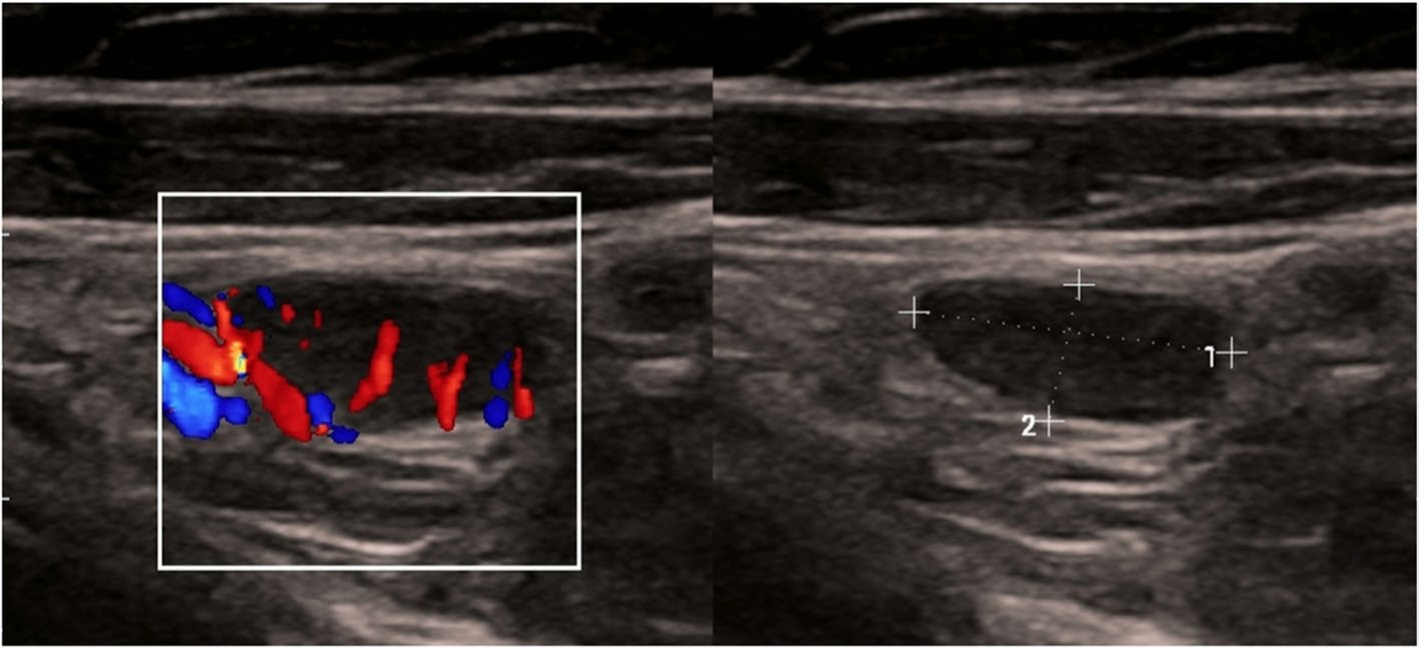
Grayscale ultrasound of the neck shows abnormal-looking lymph nodes evident by exaggerated hypoechoic echotexture and lost fatty hilum. The largest one 18 × 10 mm in diameter
